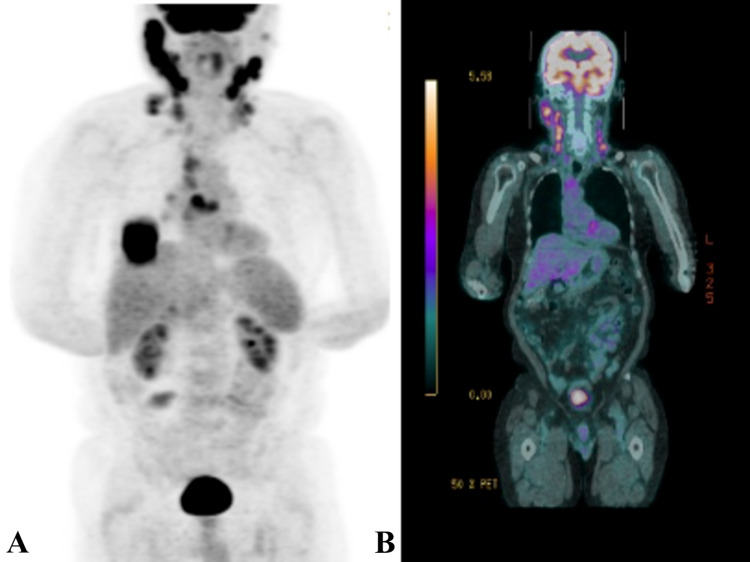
Imaging at diagnosis revealed multiple hot spots reflecting active disease (A) Positron emission tomography (PET) findings at time of ENKTL diagnosis presented as a three-dimension (3D) reconstruction revealing multiple hot spots reflecting active disease. (B) PET-computerized tomography (PET-CT) findings at time of ENKTL diagnosis revealing slice-by-slice sites of active disease. ENKTL, Extra-nodal natural killer T-cell lymphoma.
